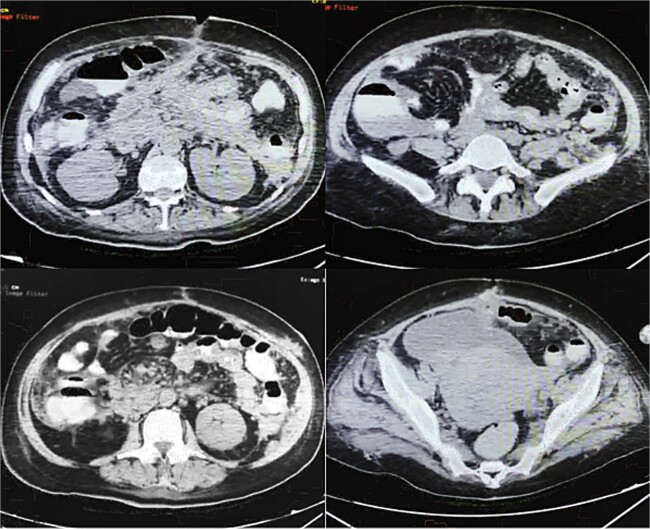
Oral contrasted abdominal CT with presence of abundant pancreatic collections on body and tail of pancreas with a volume of 132 ml, with evident fistulous tract from pancreatic parenchyma to abdominal fat and muscles near the surgical wound, torsion of mesenteric fat and vessels and terminal ileum loops in right abdominal space, free abdominal fluid on pelvic space.
